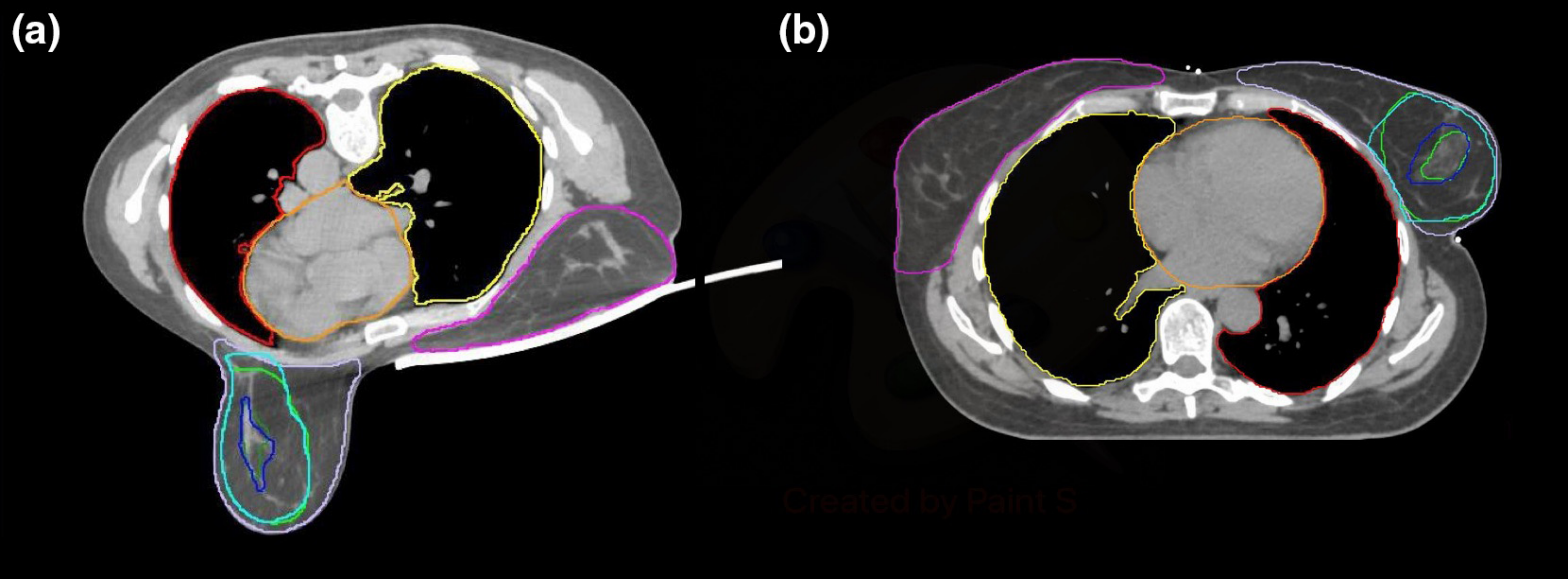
Typical CT image sets and contour information available for this investigation. A: Prone, transverse view B: corresponding transverse view, Red contour: Ipsilateral lung, Yellow contour Contralateral Lung, Orange contour: Heart, Pink contour: Contralateral Breast, Lilac contour: Ipsilateral Breast, Dark Blue contour: Seroma Cavity defined on MRI, Dark Green contour: Seroma Cavity defined on CT, Light Blue contour: planning target volume used for evaluation based off MRI seroma cavity, Light Green contour: planning target volume used for evaluation based off CT seroma cavity.
